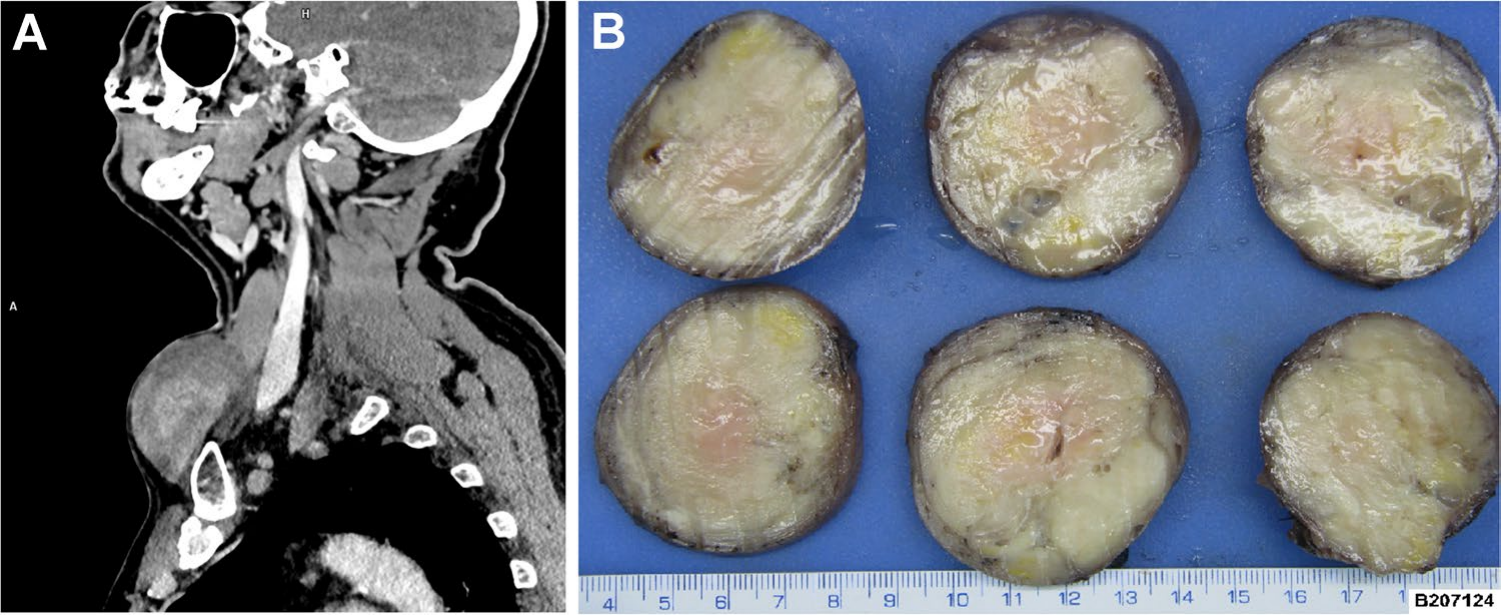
Gross and imaging features. Computed tomography showed a lower neck homogenous mass within the left supraclavicular area near the upper margin of the clavicle which was in close proximity to the tumor (A). Grossly, the tumor was oval, well-circumscribed, surrounded by a thin capsule measuring 6 cm in the largest diameter. Cut sections revealed a white to yellowish solid homogenous mass, with fibrillary arrangement containing small cystic-like areas (B)
